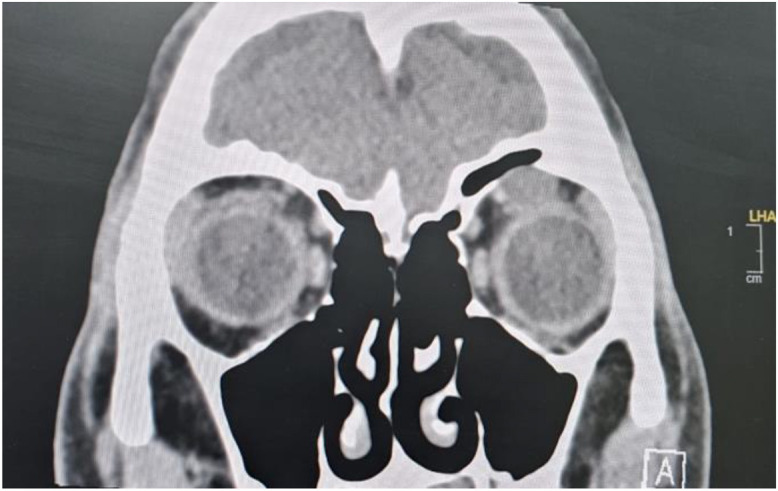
Representative CT images of the lesion.
